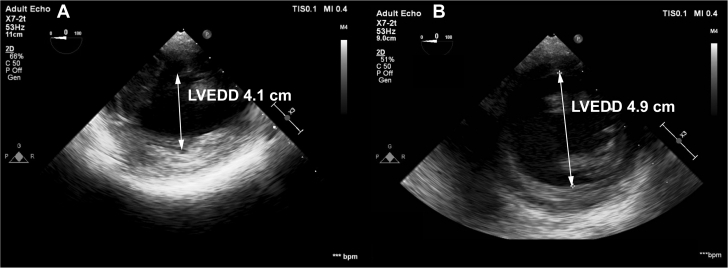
Transesophageal Echocardiography Showing Increased LVEDD (A) Left ventricular end-diastolic diameter (LVEDD) with the outflow graft unclamped. (B) LVEDD distention after outflow clamping.
